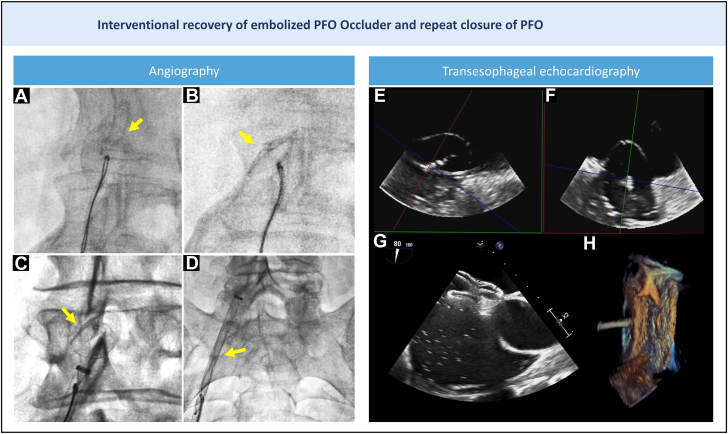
Angiography and TEE Guidance for Embolized Occlude Recovery and Repeat PFO Closure (A to D) Device (arrows) mobilization and retraction into a 14-F sheath by snaring with a tulip snare inside a Judkins right 6-F catheter. (E and F) A stretched diameter of 22 mm was measured by balloon sizing. (G) Complete abolition of right-left shunt as demonstrated by bubble testing. (H) Three-dimensional TEE showing adequate device positioning. PFO = patent foramen ovale; TEE = transesophageal echocardiography.
